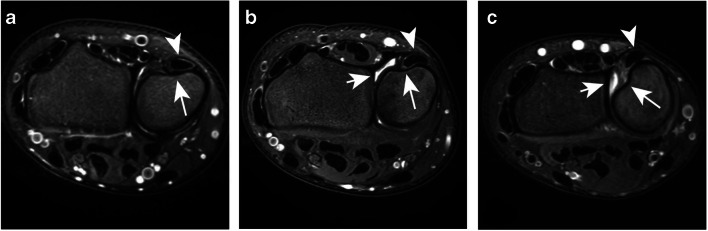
Axial T2 weighted fat-saturated MRI showing the ECU tendon (arrowheads) position a completely within, b partially within, and c completely outside its groove (long arrows). All three wrist positions were scored as a supinated wrist position with the ulnar head in dorsal rotation. Image b and c also show a substantial amount of DRUJ effusion (short arrows)
